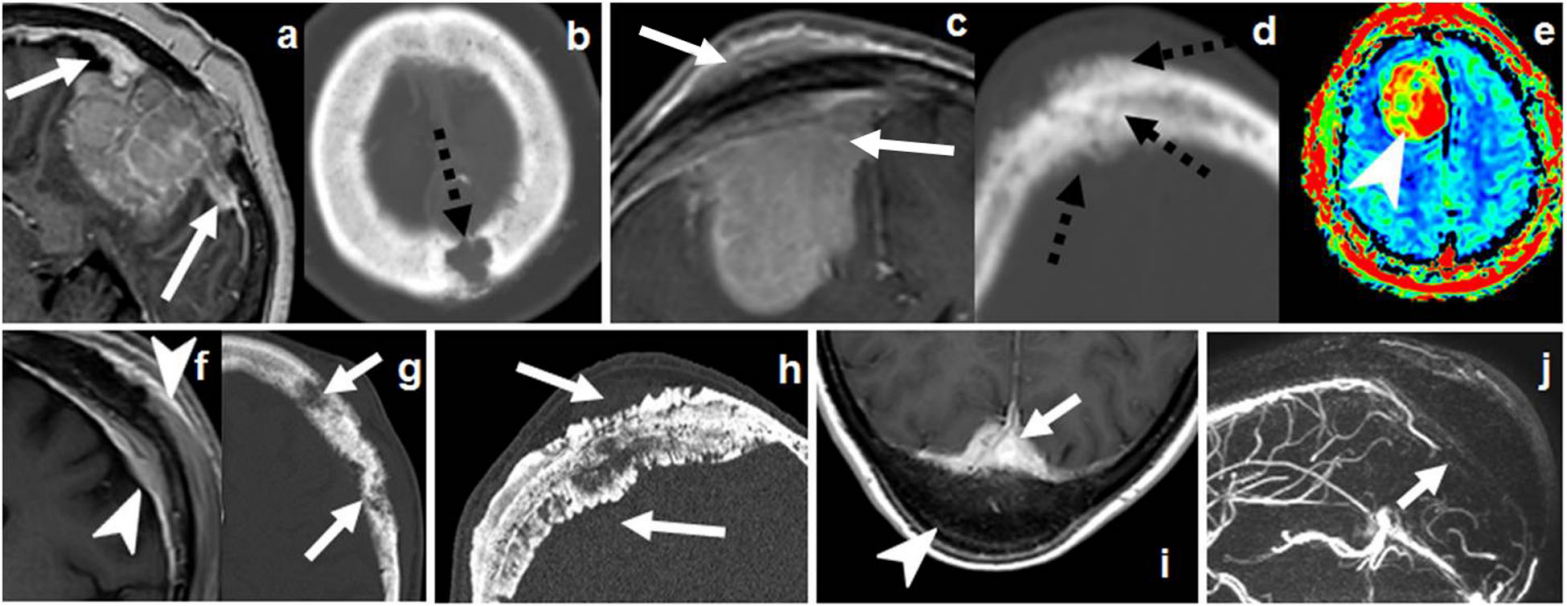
Meningioma. Great polymorphism. Case 1 (a, b): Post-contrast T1WI (a) and CT (b): dural-based with dural tails (arrows) and bone lysis (dashed arrows). Case 2 (c–e): Post-contrast T1WI (c), CT (d), and DSC-PWI (e): dural-based hypervascular (arrowhead) with transdiploic growing (arrows), bone sclerosis-permeation, and aggressive-like periosteal reaction (dashed arrows). Case 3 (f, g): Post-contrast T1WI (f) and CT (g): diploic-centered plaque-like with intra- and extracranial laminar soft-tissue (arrowheads) and bone lysis-permeation (arrows). Case 4 (h): CT shows diploic-centered lesion with great spiculated hyperostosis (arrows). Case 5 (i, j) Post-contrast T1WI (i) and MR venography (j): diploic-centered with great homogeneous smooth hyperostosis (arrowhead) and intracranial soft-tissues characteristically invading the superior longitudinal venous sinus (arrows)
